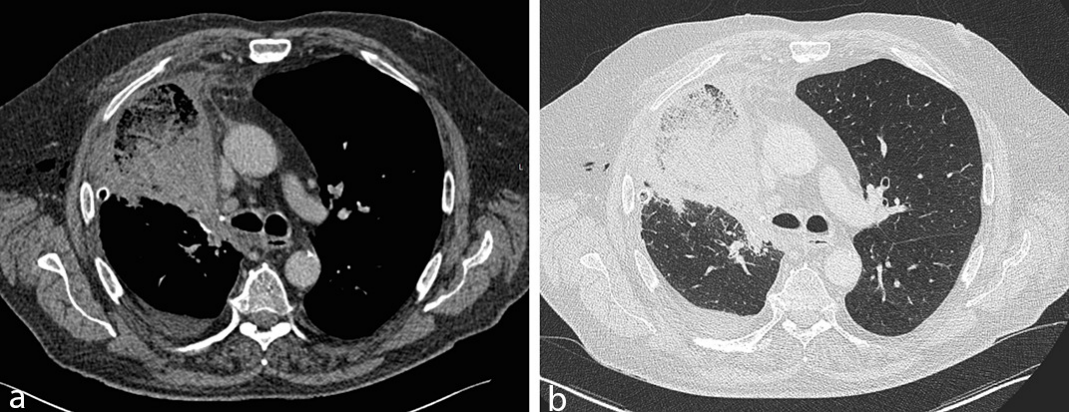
Axial images from the post-operative contrast-enhanced CT scan. (a) Soft tissue window shows an expanded, poorly enhancing right middle lobe, which tapers towards the hilum. (b) Lung window demonstrates extensive ground-glass opacity, consolidation and interlobular septal thickening.
